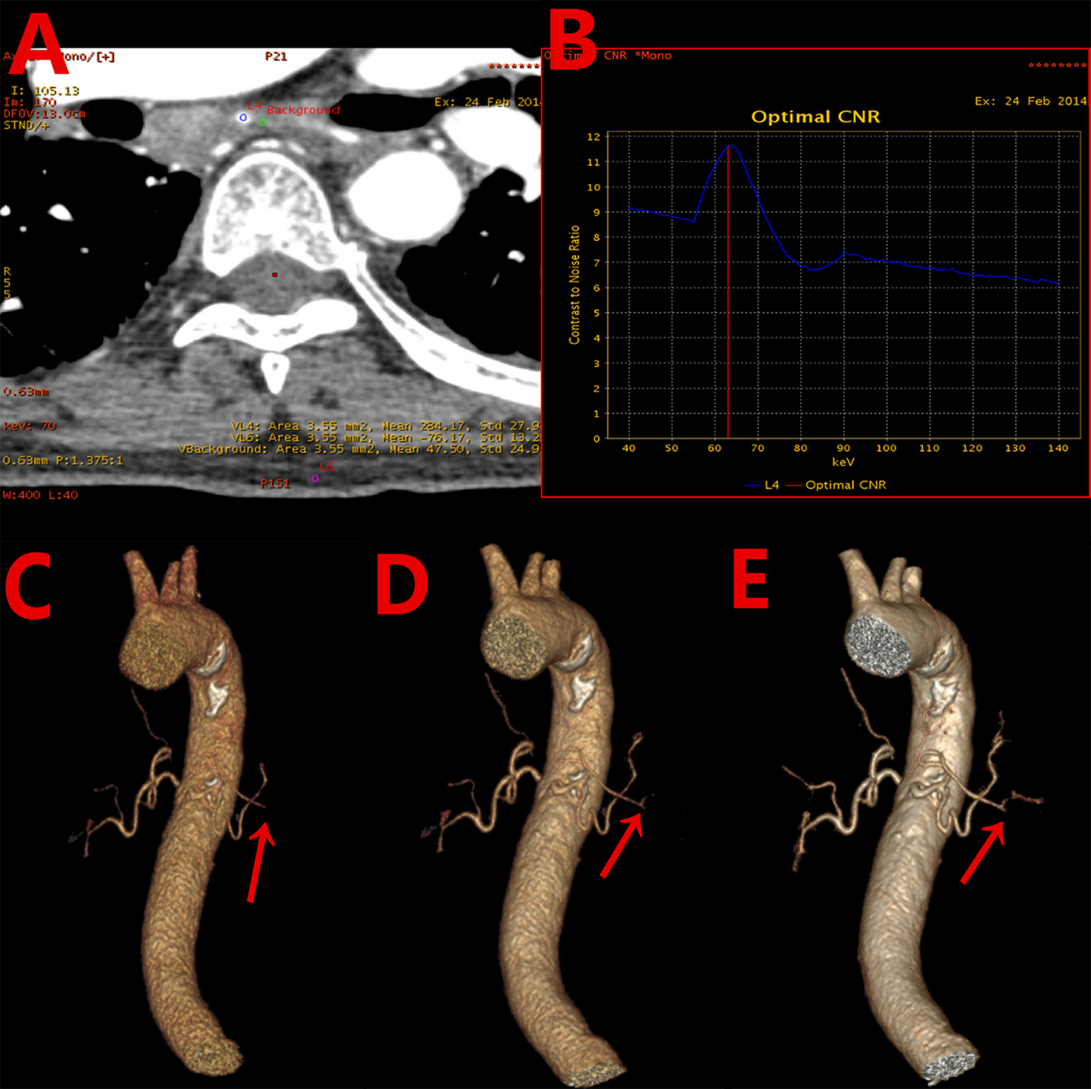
A 68 years old male patient with cancer in the right lung. (A) Axial image with region-of-interest indications for measuring CT number and standard deviation: bronchial artery blood vessels (blue), adjacent tissues in mediastinum (green), and subcutaneous fat on chest wall (purple). (B) Plot of contrast-to-noise ratio(CNR) as function of photon energy showing the optimal energy level of 63keV to obtain the highest CNR for the bronchial artery. (C) Volume-rendering (VR) 140kVp image with image quality score of 3. (D) VR image at 70 keV with image quality score of 4. (E) VR image at the optimal 63 keV with image quality score of 5.
